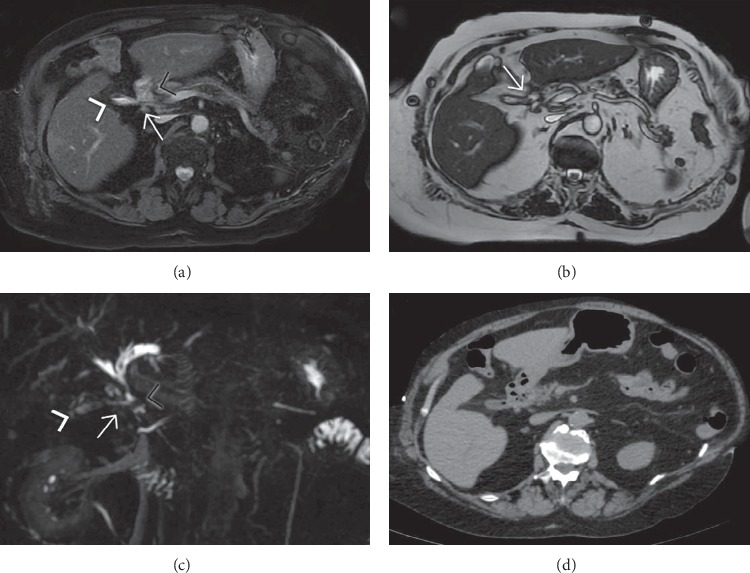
Magnetic resonance imaging, fat-suppressed FIESTA axial sequence (a), SSFSE T2-weighted axial sequence (b), MIP-reformatted 3D-MRCP sequence (c), and unenhanced computed tomography of a patient with iodinate contrast media allergy treated for gallstone ileus with enterolithotomy. The fistulous communication (arrow in (a), (b), and (c)) between the gallbladder (white arrowhead in (a), (b), and (c)) and the duodenum (black arrowhead in (a), (b), and (c)) is readily visible in the MR images due to the presence of a small amount of fluid in its collapsed lumen. The CT study (d) could not provide an accurate fistula anatomy required by the abdominal surgeon to decide for a possible fistula repair.
